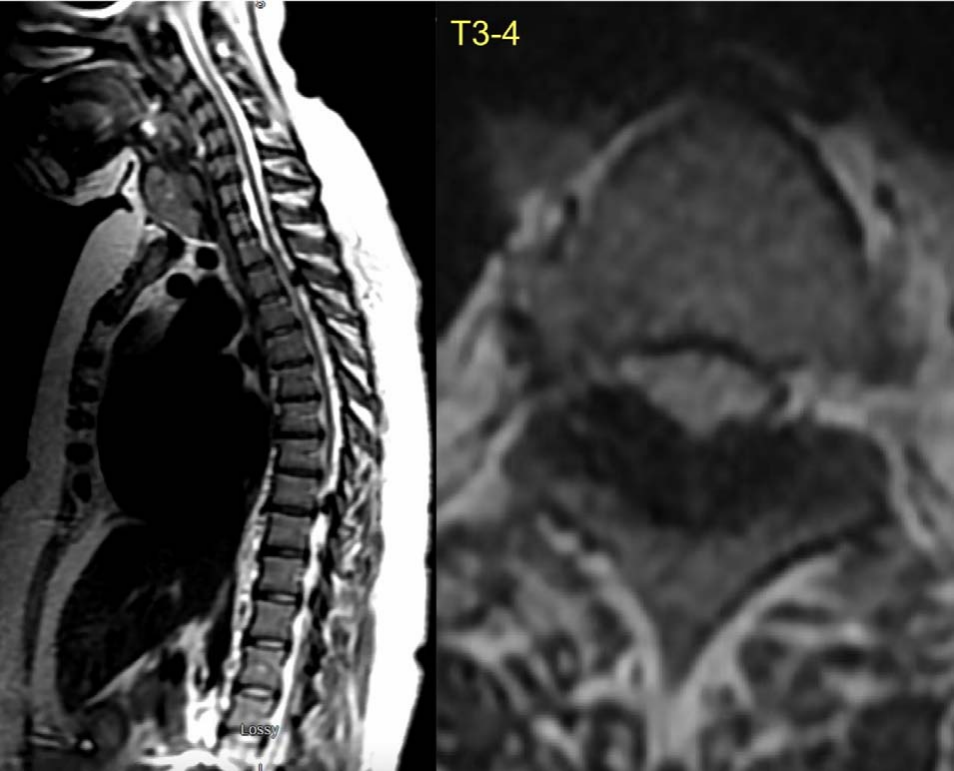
T2 magnetic resonance imaging cuts (left) showing multiple segmental calcifications of the ligamentum flavum throughout the thoracic spine, with severe spinal stenosis at the T3-T4 level (right).
